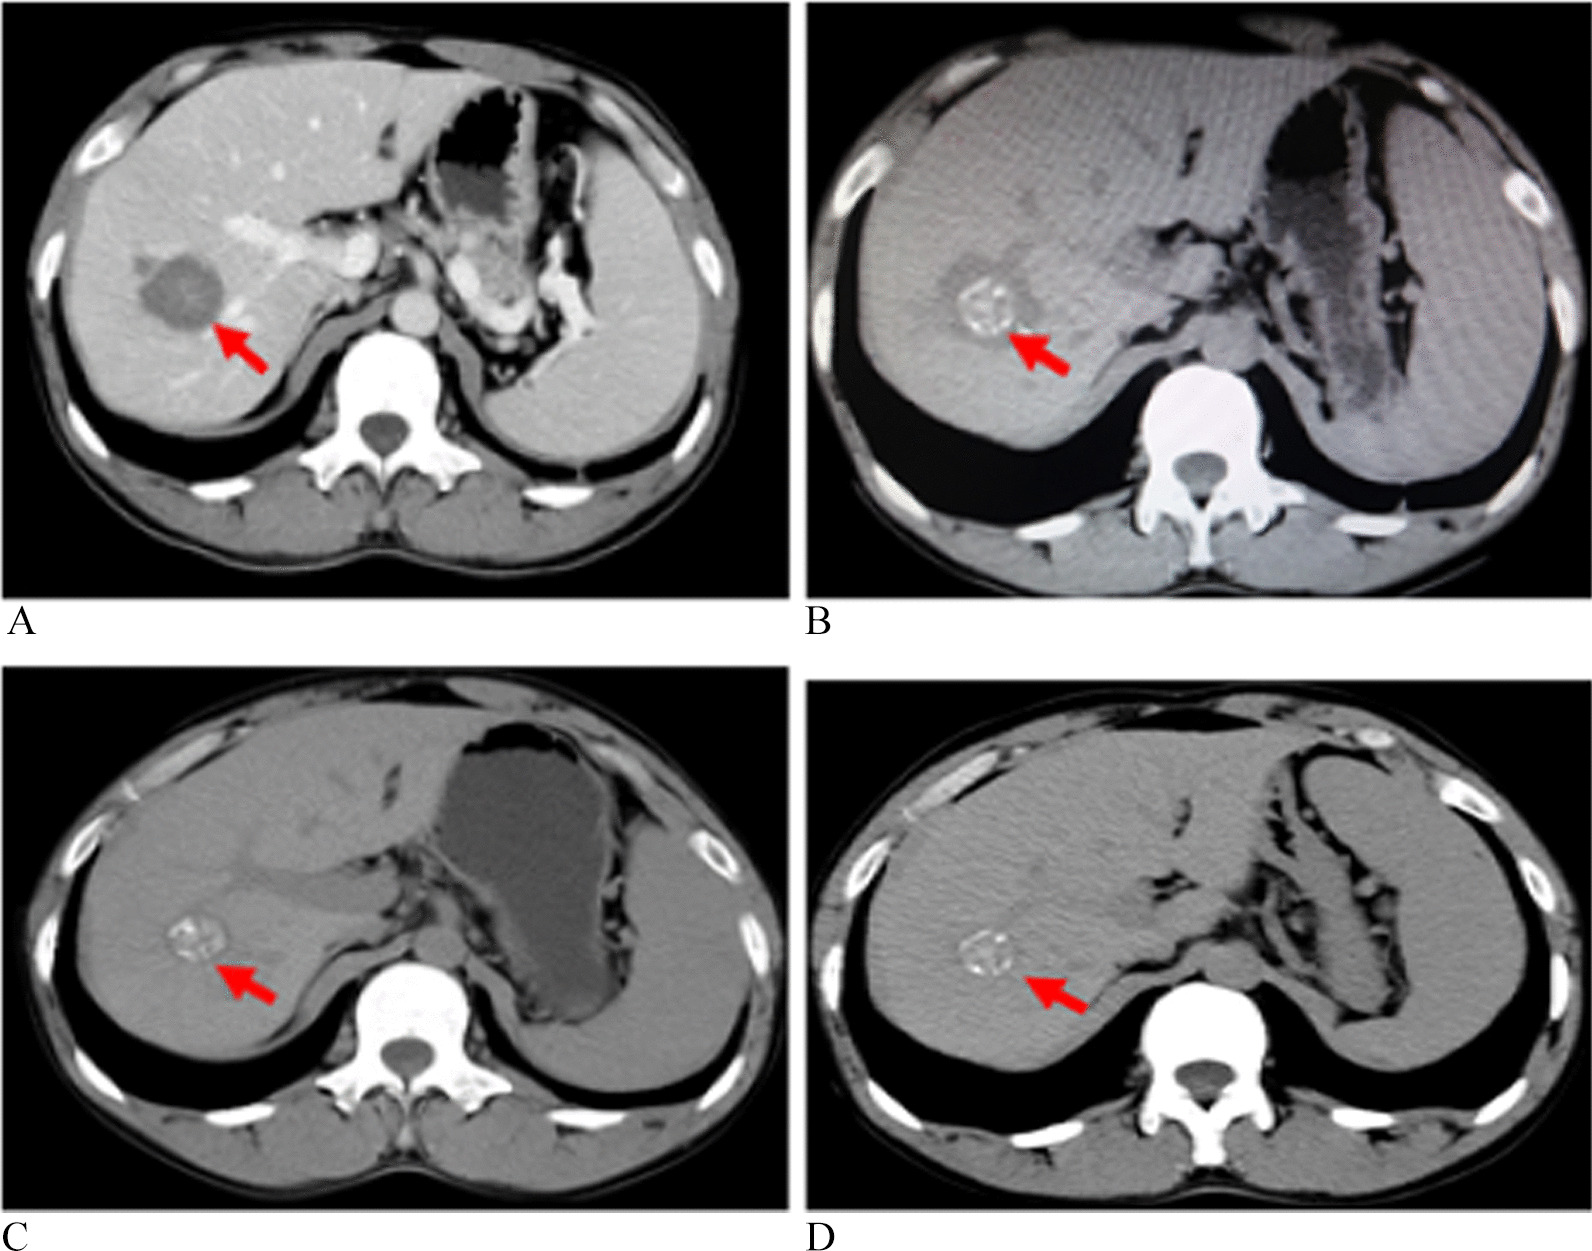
Images showing the lesions before and 1, 6, 12 months after MWA in a 36-year-old male with HAE. A Enhanced CT was performed before ablation. B CT scan 1 month after ablation. C CT scan 6 months after ablation. D CT scan 12 months after ablation. The red arrow indicates the HAE lesion. HAE, hepatic alveolar echinococcosis; MWA, microwave ablation
